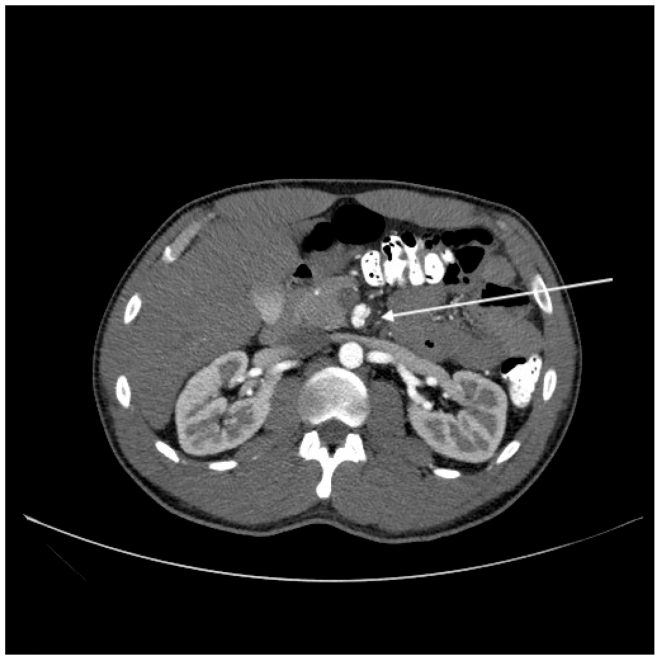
Preoperative computed tomography (CT) scan showing evidence of dissection flap approaching the take-off of the colic arteries. *Arrow* points to superior mesenteric artery (SMA) with dissection.
